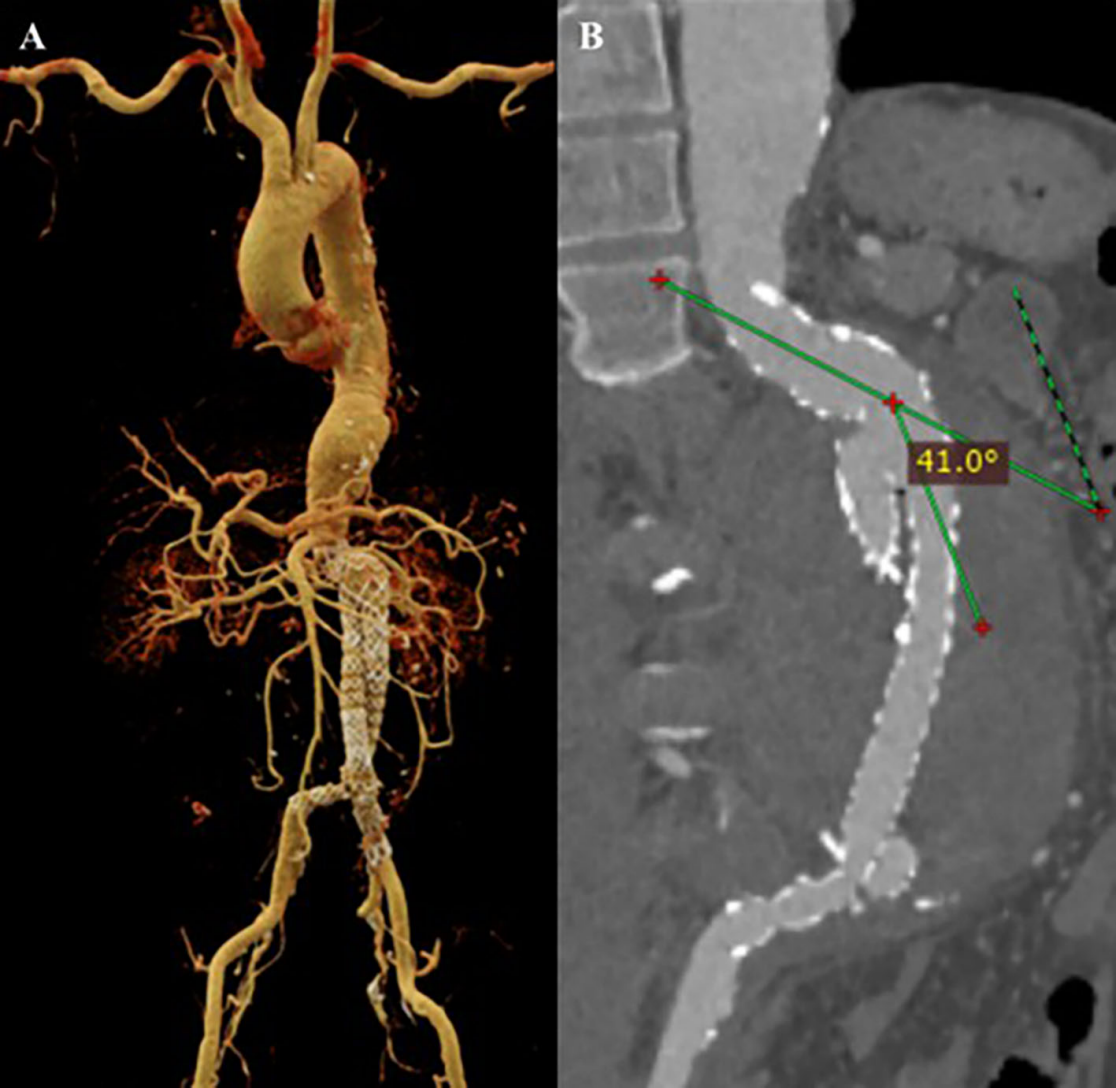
CT Scan after EVAR procedure. A. volume rendering shows the stent graft position with no evidence of endoleak; B. Maximum Intensity Projection of CT scan shows anatomy features of the conical neck and angulation of the abdominal aorta.
